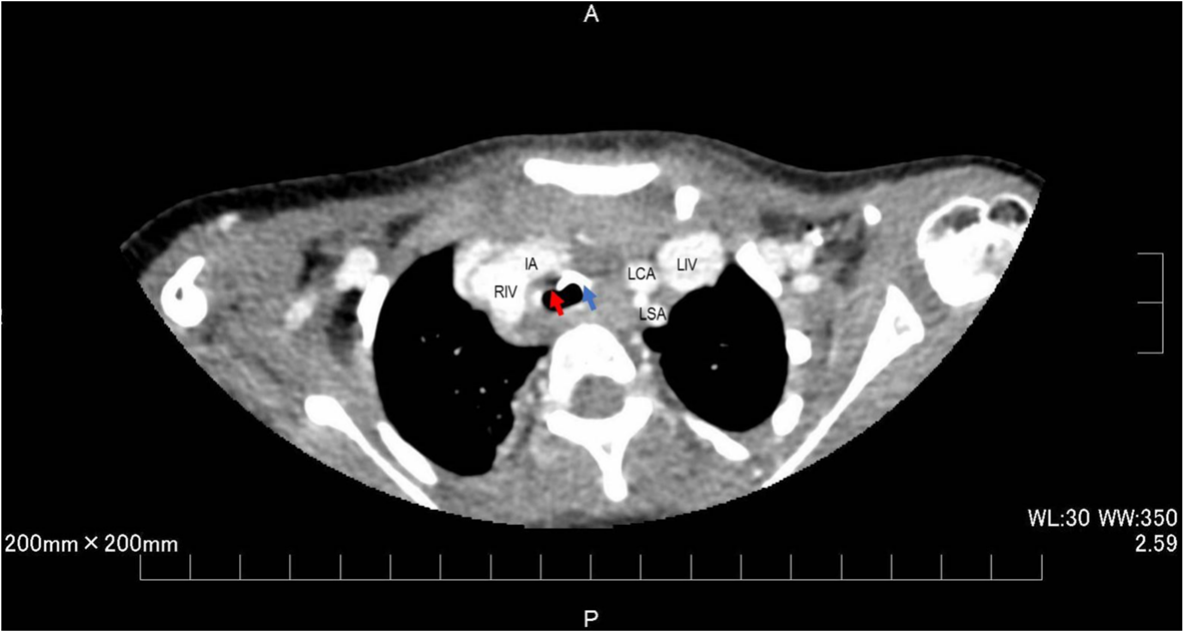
Preoperative contrast-enhanced computed tomography. Computed tomography demonstrated the innominate artery was in contact with the trachea. Red and blue arrow shows tracheo-innominate artery fistula and the tip of cannula, respectively. IA; innominate artery, RIV; right innominate vein, LCA; left common carotid artery, LSA; left subclavian artery, LIV; left innominate vein
